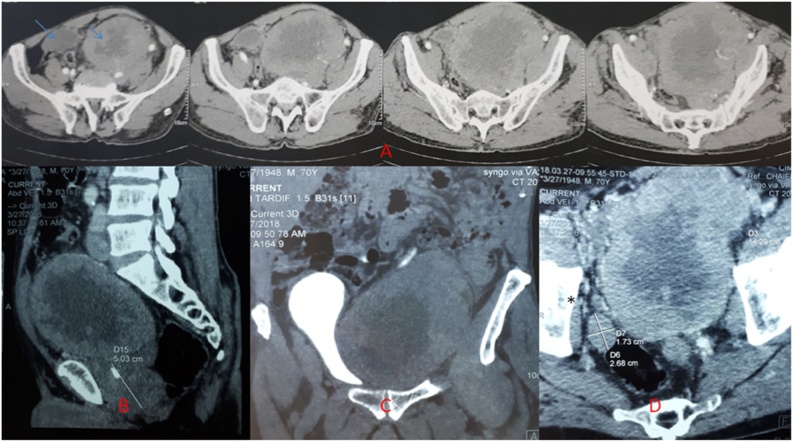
CT scan images showing a large lobulated necrotic pelvic and extraperitoneal mass with multiple swollen lomboaortic and iliac lymph nodes.
